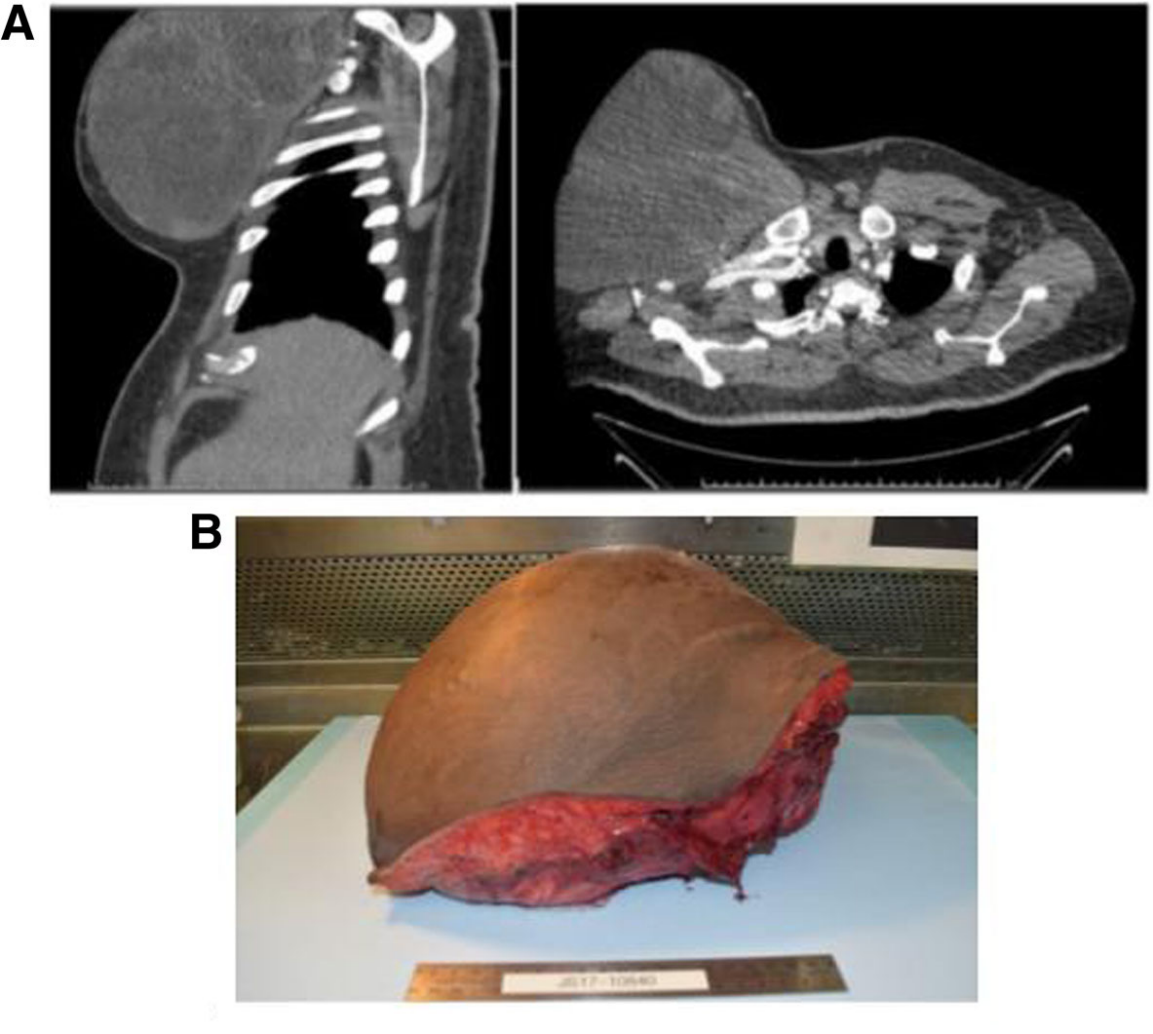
a Preoperative CT Chest b En bloc resection chest wall sarcoma
